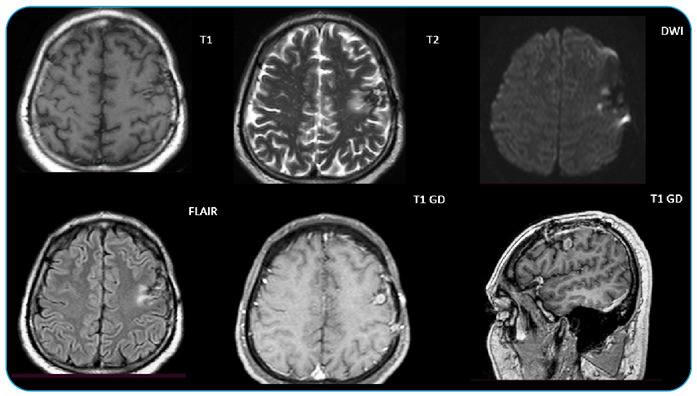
After the second month of amoebic brain abscess treatment. Left to right, top to bottom, brain MRI sequences of axial slices in unenhanced T1, T2, diffusion, FLAIR, T1 with contrast, and sagittal T1 with contrast, respectively. Radiological improvement after treatment demonstrated by blue arrows.
